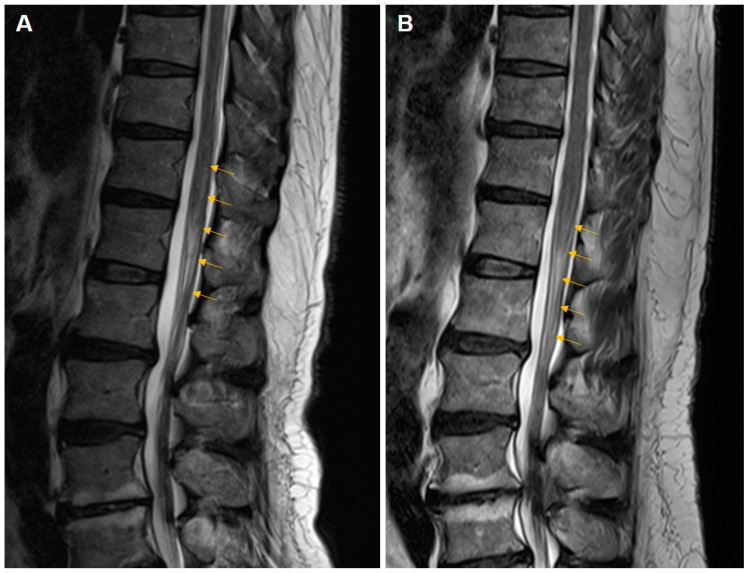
Sagittal T2-weighted views from thoracolumbar spinal magnetic resonance imaging (MRI). MRI at the first attack showed a diffuse longitudinal high-signal lesion (yellow arrows) with mild enlargement of the spinal cord from T12 to L1 (A). MRI at the recurrent attack revealed a similar lesion from L1 to L2 (B).
